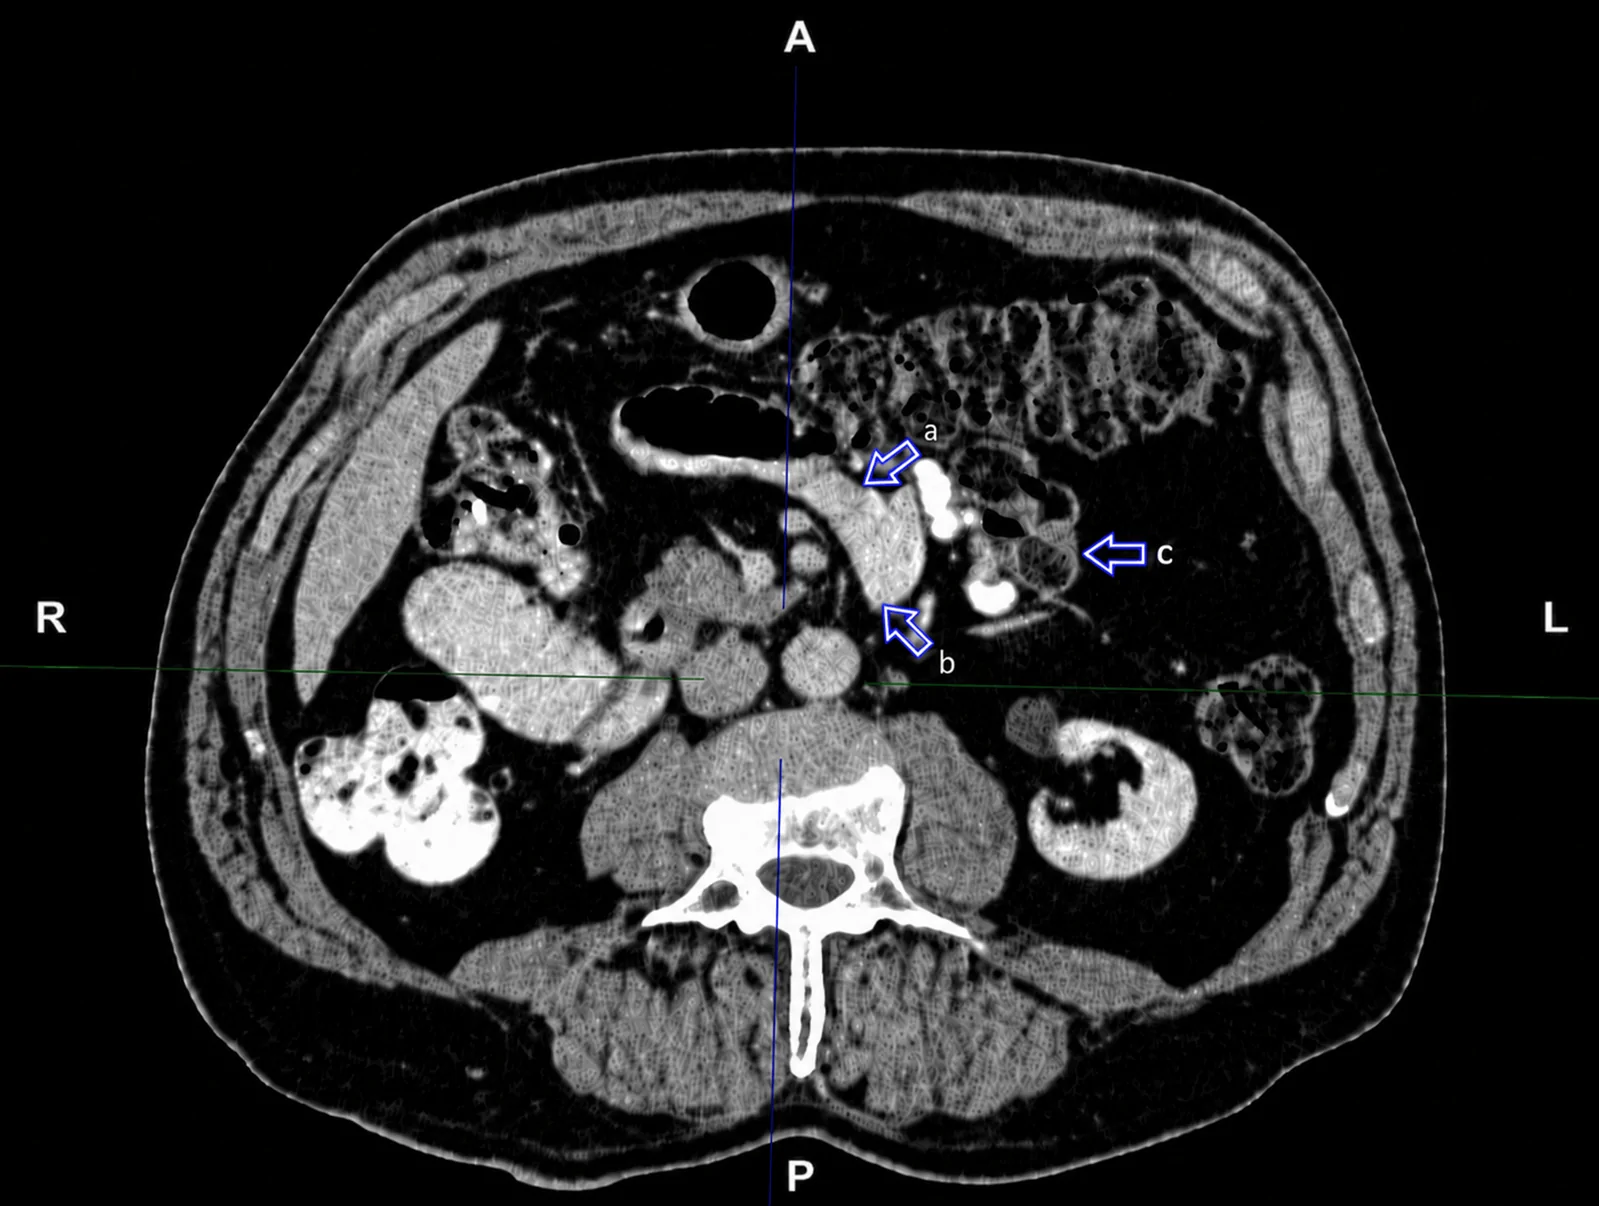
Axial contrast-enhanced CT image of patient 3. Abnormal configuration and course of the duodenum. A small diverticulum is present in the medial part of the bulb, and just below it, there is a relatively narrow passage to the distal part of the duodenum (a), resembling a diverticulum at the site of the passage (b). The lower bend and horizontal part of the duodenum are located low, anterior to the origin of the inferior mesenteric artery from the aorta. The first loop of the jejunum passes to the right side at approximately the level of the pancreas (c). The loops of the small intestine within the scope of the examination are mostly located on the right side, while part of the ileum is located in the left lower abdomen, features consistent with malrotation. R, L, A, and P indicate the patient’s right, left, anterior, and posterior sides, respectively. CT: computed tomography
